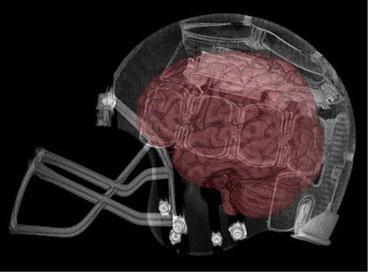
CT scan of an instrumented helmet merged with an MRI of a human brain demonstrating how the accelerometer array fits between the normal padding of football helmets
